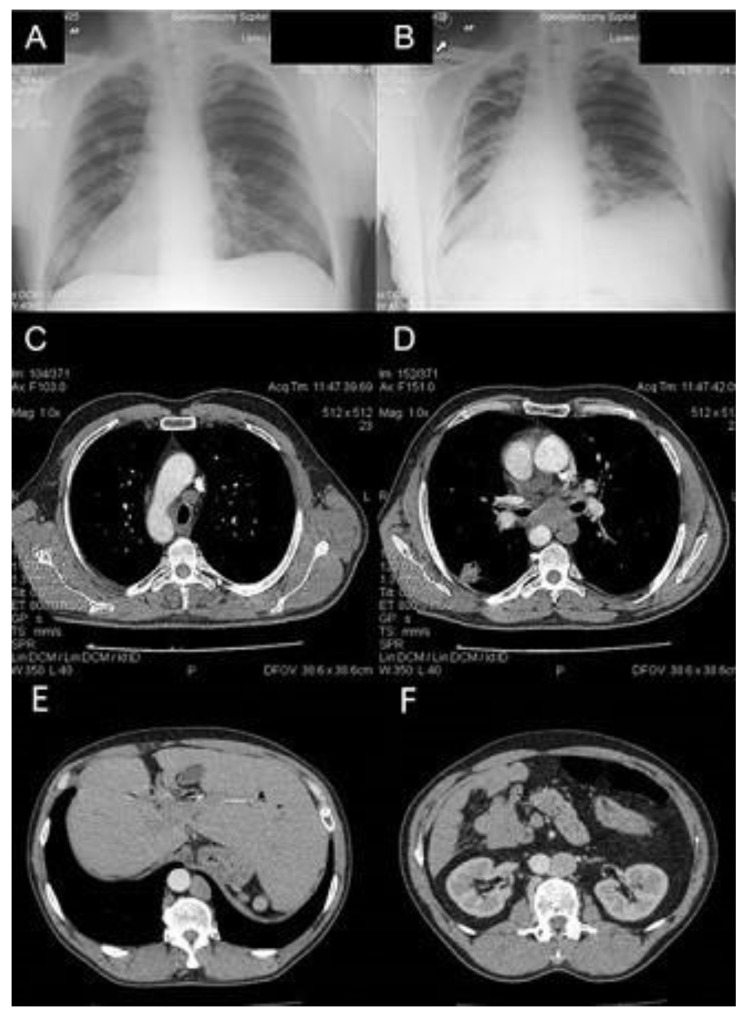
(A,B) Pre- and postoperative chest X-rays of the patient with SIT and lung cancer. (C,D) Chest CT showing rearrangement of mediastinal vessels and 2.5 cm tumor in VI right segment with enlargement of lymph nodes 4R and 7. (E,F) Abdominal CT showing position of the liver on the left side and position of the aorta on the right side.
